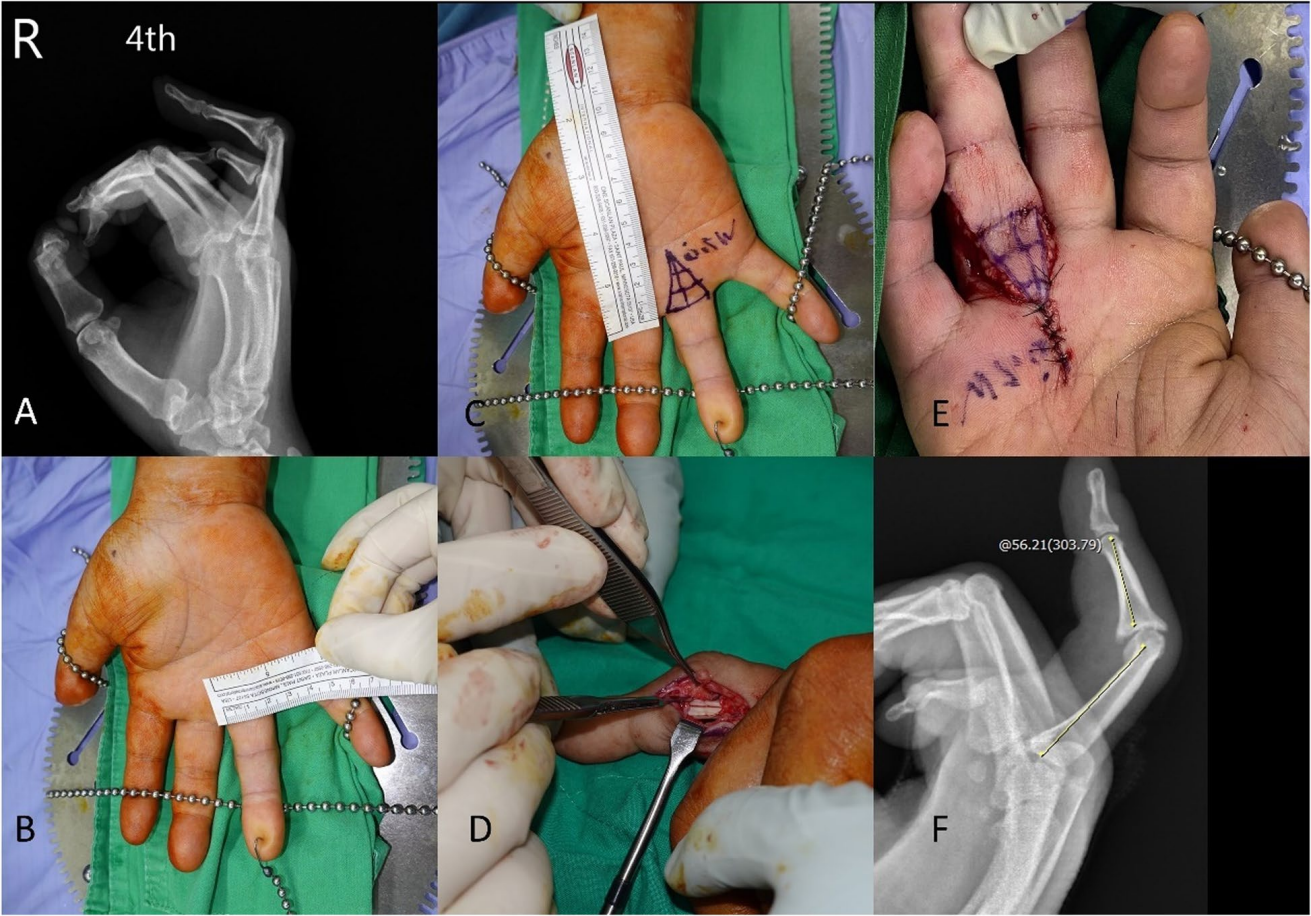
A. The extension lag of the patient’s PIP joint was 83°. B. The width of the digit base (W) was 20.0 mm. C. The height of the V–Y flap is 1.5 W from the base of the contracted digit, which is 30.0 mm. D. Tenoarthrolysis performed through bilateral mid-lateral approach. E. Full passive extension of the contracted digit, recorded during the surgery. F. Postoperative plain film showing that the extension lag of right fourth PIP joint has decreased to 56° actively
